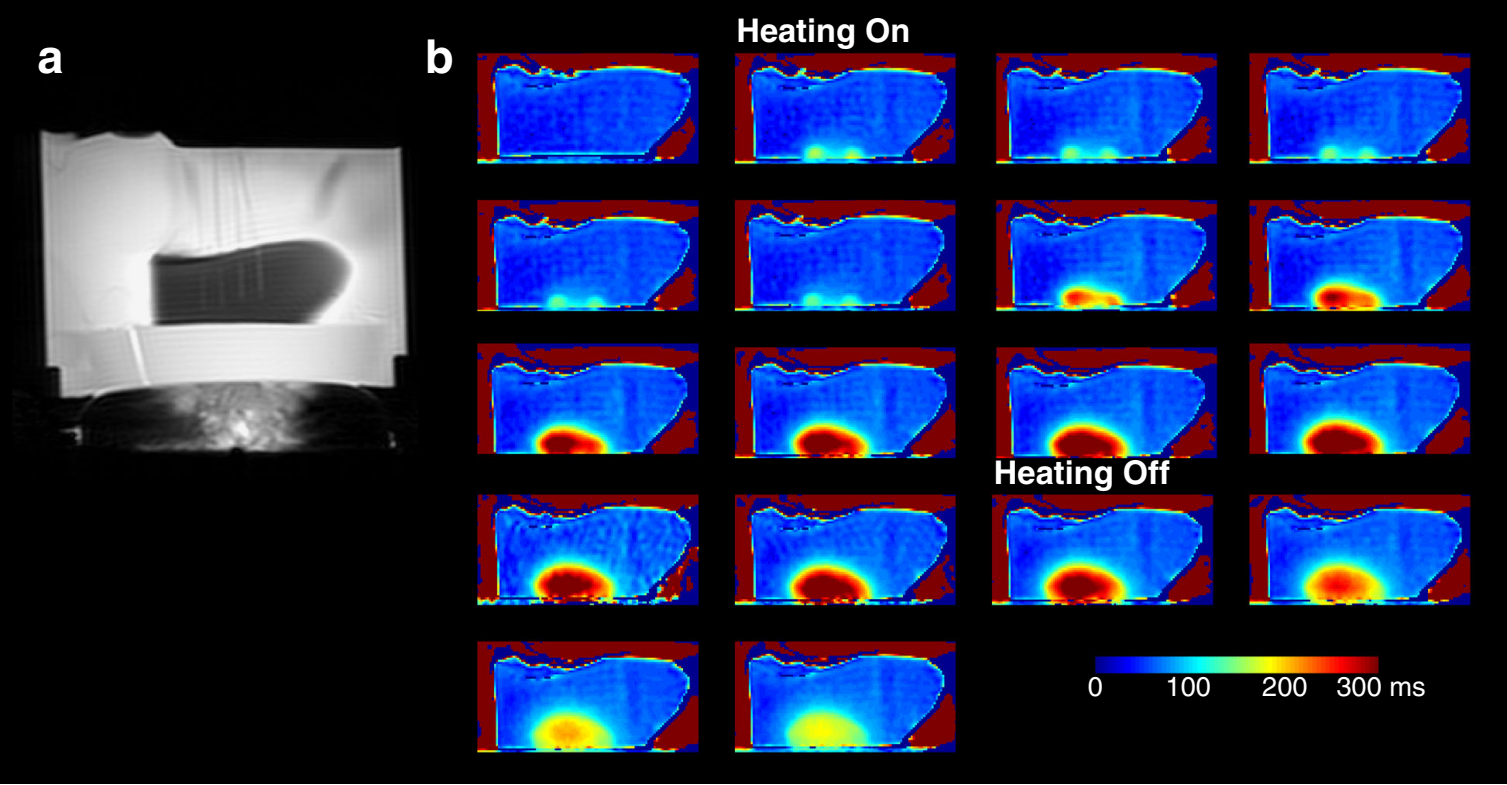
a Magnitude image acquired during sonication showing the setup for T2-based thermometry of trabecular bone marrow during focused ultrasound sonications showing the fiber optic probes; b T2 maps of sample 1 during heating and cool-down
